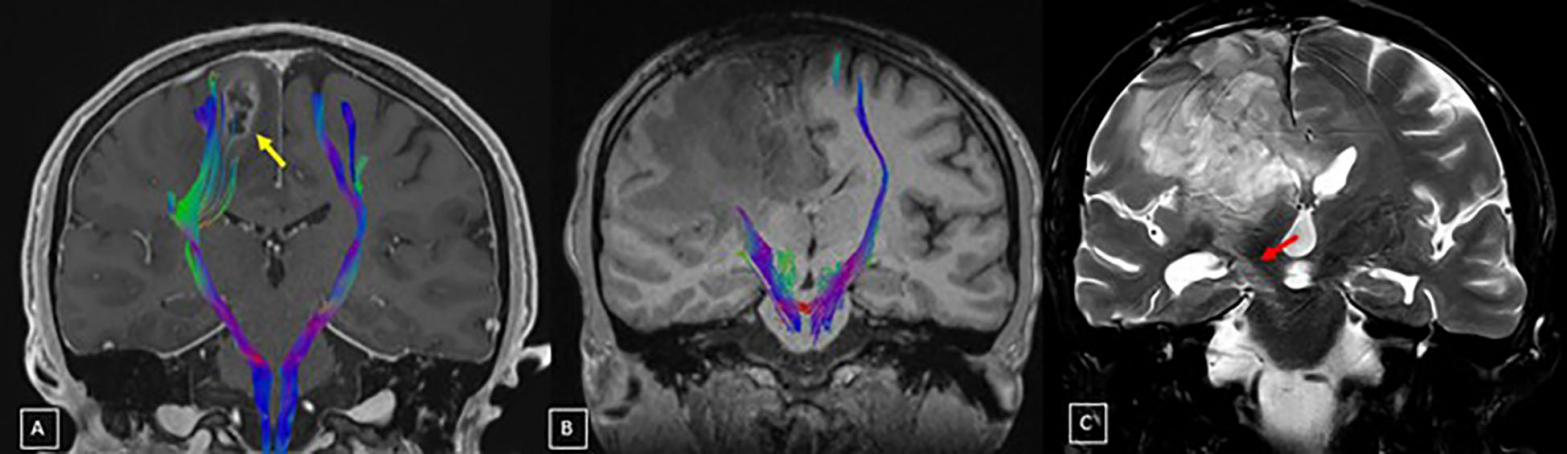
Pre-treatment baseline study (A), tractography combined with post-contrast T1-weighted MRI in the coronal plane reveals a lesion in the right frontal lobe, with a necrotic center, irregular margins, and contrast enhancement (yellow arrow). In the tractography study (A), there is asymmetry in the distribution of fractional anisotropy in the signal columns representing the right corticospinal tract, with posterior displacement. After treatment, a marked reduction in the fibers representing the topography of the right CST is noted (B). A few months after treatment, coronal plane T2-weighted MRI (C) shows a signal alteration along the course of the right corticospinal tract, extending from the posterior limb of the internal capsule to the pons, characterized by T2/FLAIR hyperintensity, without significant retractile effect, diffusion restriction, or contrast enhancement, suggestive of WDCT (red arrow).
